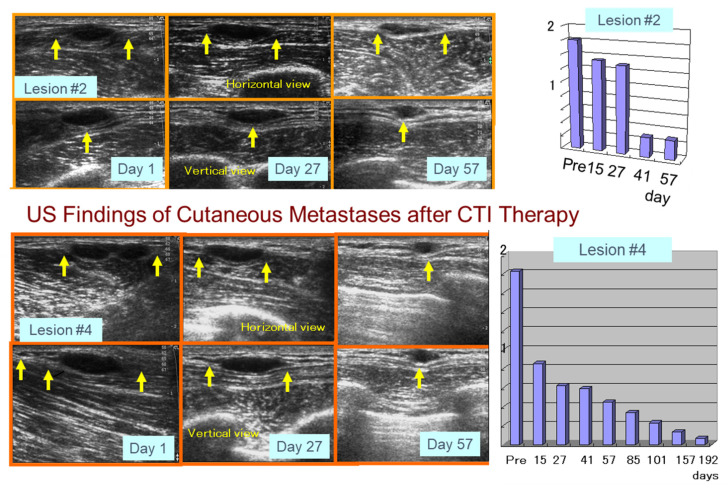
Ultrasound (US) findings of distant melanoma metastases after CTI therapy. Arrows in the photo indicate horizontal and vertical views of metastatic tumors comparing the decreased size of the tumor volume.
